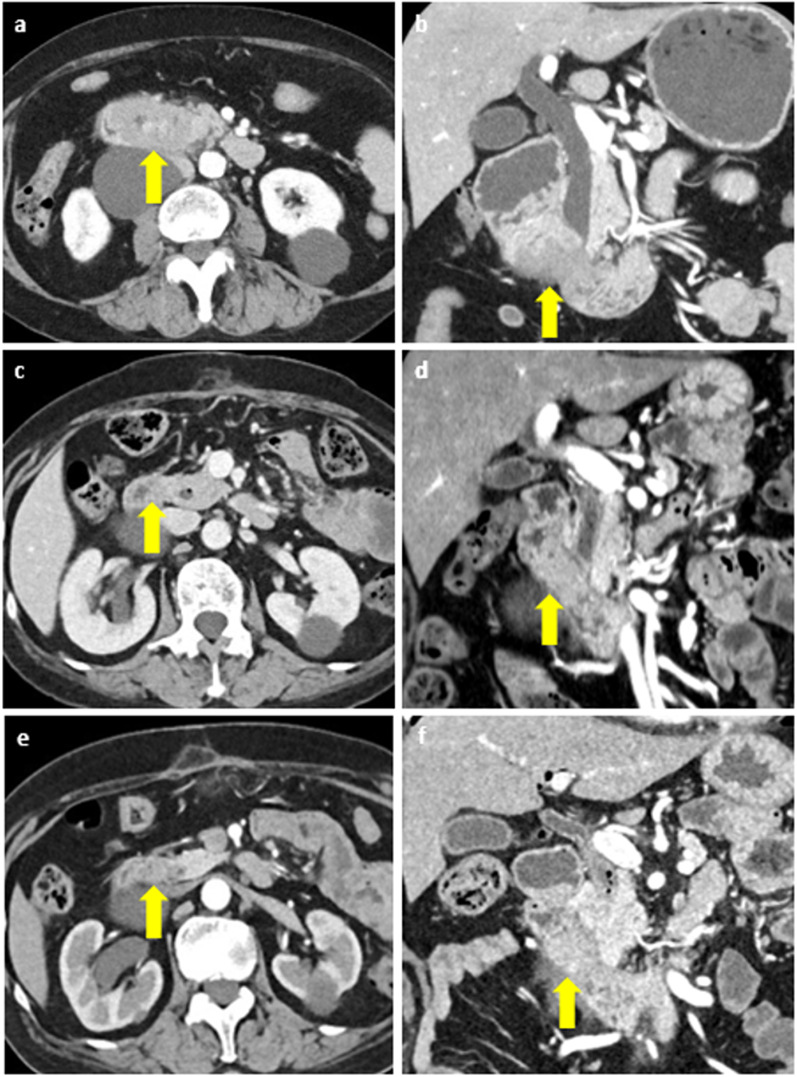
Contrast enhanced abdominal computed tomography. a Before chemotherapy, axial view. The tumor was at the second portion of the duodenum (arrow). The lumen of the duodenum and the common bile duct was almost obstructed. b Before chemotherapy, coronal view. c After 3 courses of chemotherapy, axial view. The size of the tumor had decreased (arrow). d After 3 courses of chemotherapy, coronal view. e After 6 courses of chemotherapy, axial view. The tumor had extremely shrunken and was not detected (arrow). f After 6 courses of chemotherapy, coronal view
